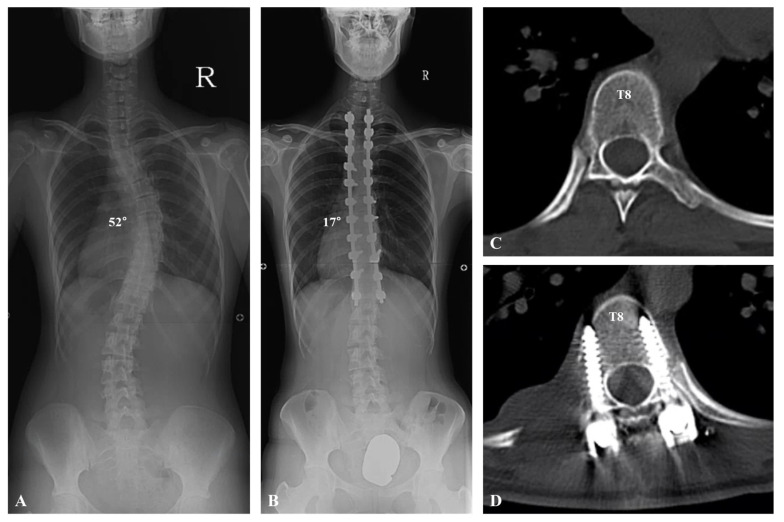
A 15-year-old female patient visited the hospital for progressive spinal deformity. (A) The whole-spine anteroposterior image showed 52° of scoliosis deformity with T8 apical vertebra. (B) Deformity correction from T2 to T12 was performed using monoaxial pedicle screws and rod derotation, producing a correction from 52° to 17°. (C,D) Axial view of computed tomography presented the apical vertebra (T8) before and after deformity correction.
